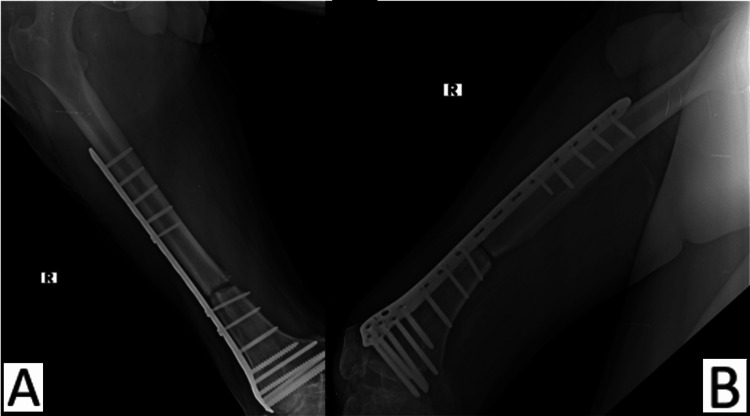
(A) Anteroposterior and (B) lateral X-ray views of distal femur plating showing nonunion (managed by exchange plating with bone graft application)
